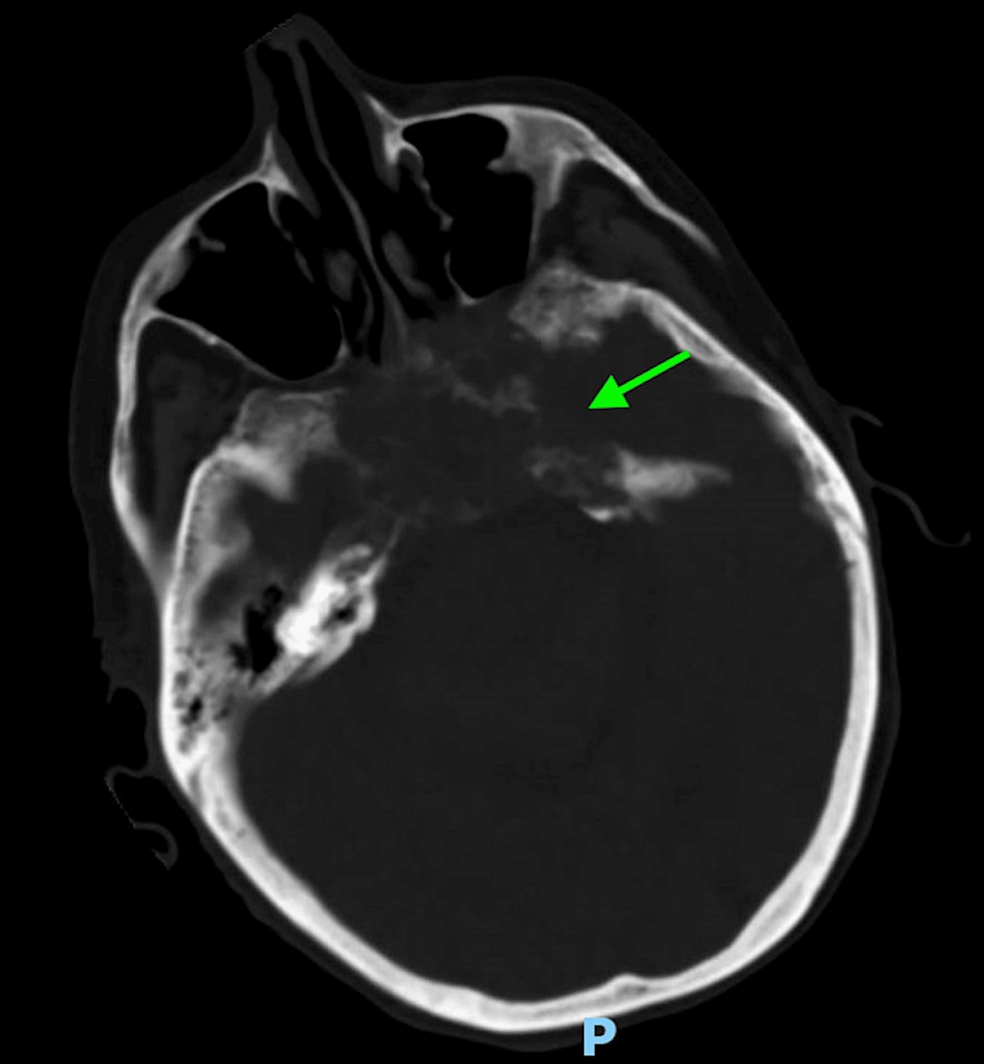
Axial image obtained from computed tomography of the head demonstrates clival chordoma with sphenoid sinus obliteration, involvement of the cavernous sinus, and bilateral petrous apices (green arrow)
